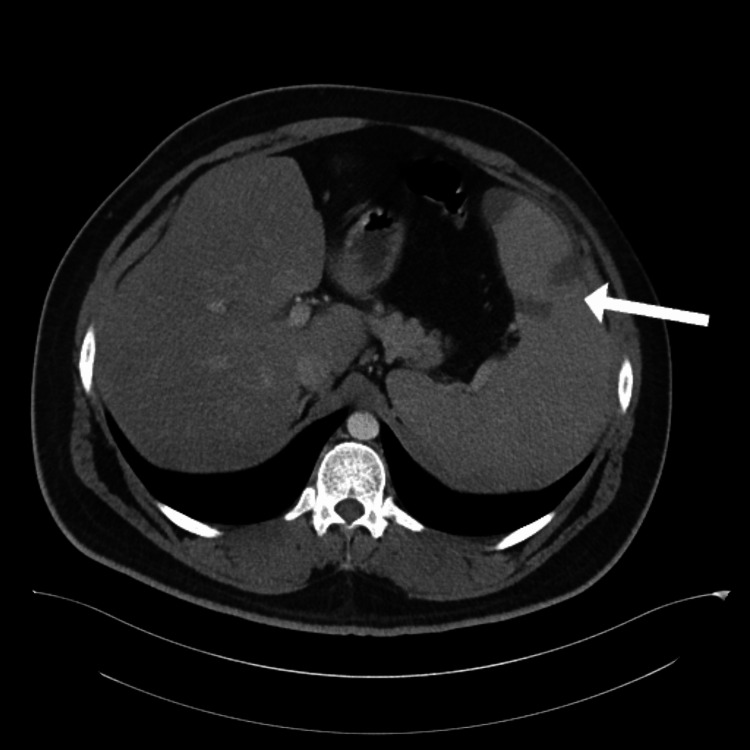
Transverse plane CT scan of abdomen showing multiple infarcts in spleen (white arrow)
